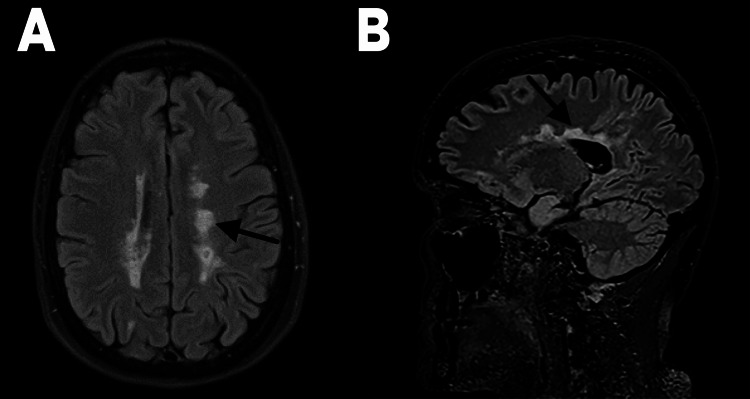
(A, B) FLAIR MRI performed during 2019 seizure admission for seizure activity showing periventricular hyperintensities (black arrows) consistent with her prior 2018 MRI images.
